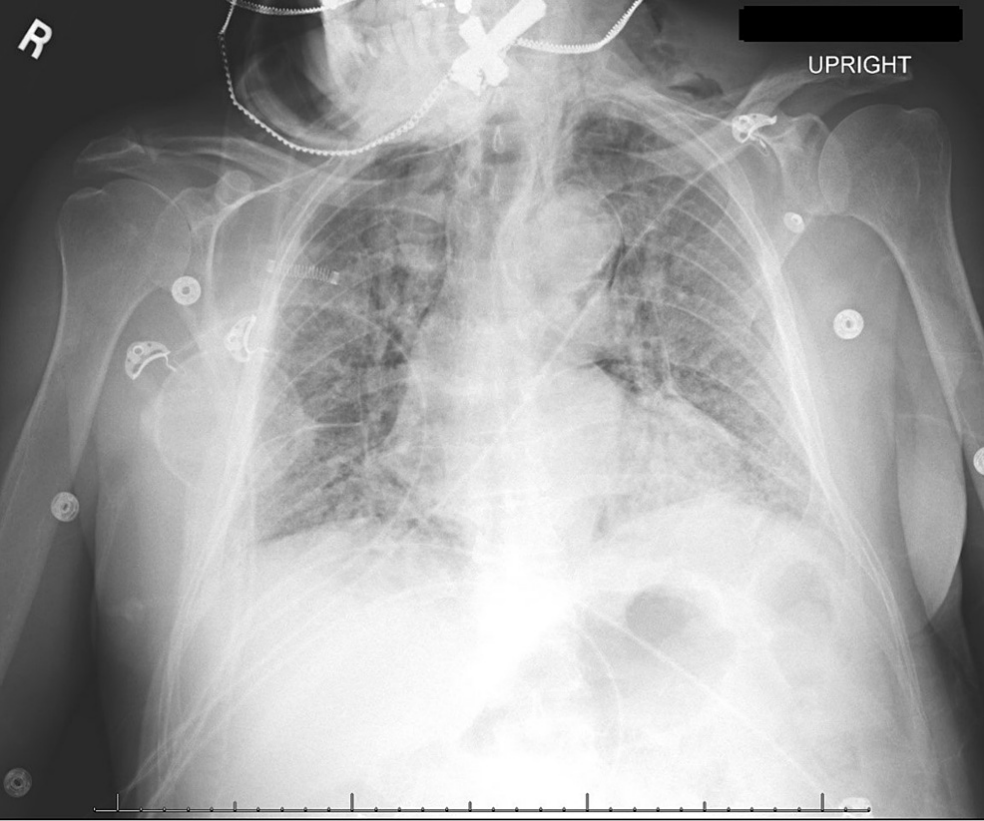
Chest X-ray day 10 of admission revealing extensive bilateral infiltrates with pneumomediastinum and subcutaneous emphysema.
